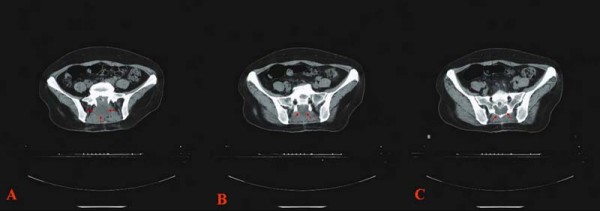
Computed tomography (CT) scan of the abdomen and plevis demonstrated a small (2 × 1.5 × 0.8 cm) low-density, well circumscribed mass in the sacrum, without evidence of tumor infitration of adjacent structures (three different CT-slice, A, B, and C).
